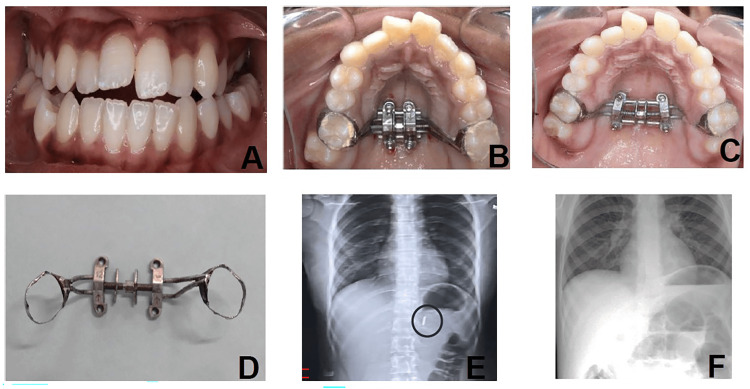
Case 1: Accidental swallowing of the MSE connecting rod. (A) Transverse maxillary deficiency with bilateral crossbite. (B) Insertion of MSE with four screws. (C) Midline diastema with MSE. (D) Autodetached MSE connecting rod. (E) Abdominal radiograph immediately after swallowing showing a swallowed connecting rod. (F) Seven-day post-ingestion abdominal radiograph confirming the absence of the connecting rod. MSE: maxillary skeletal expander
